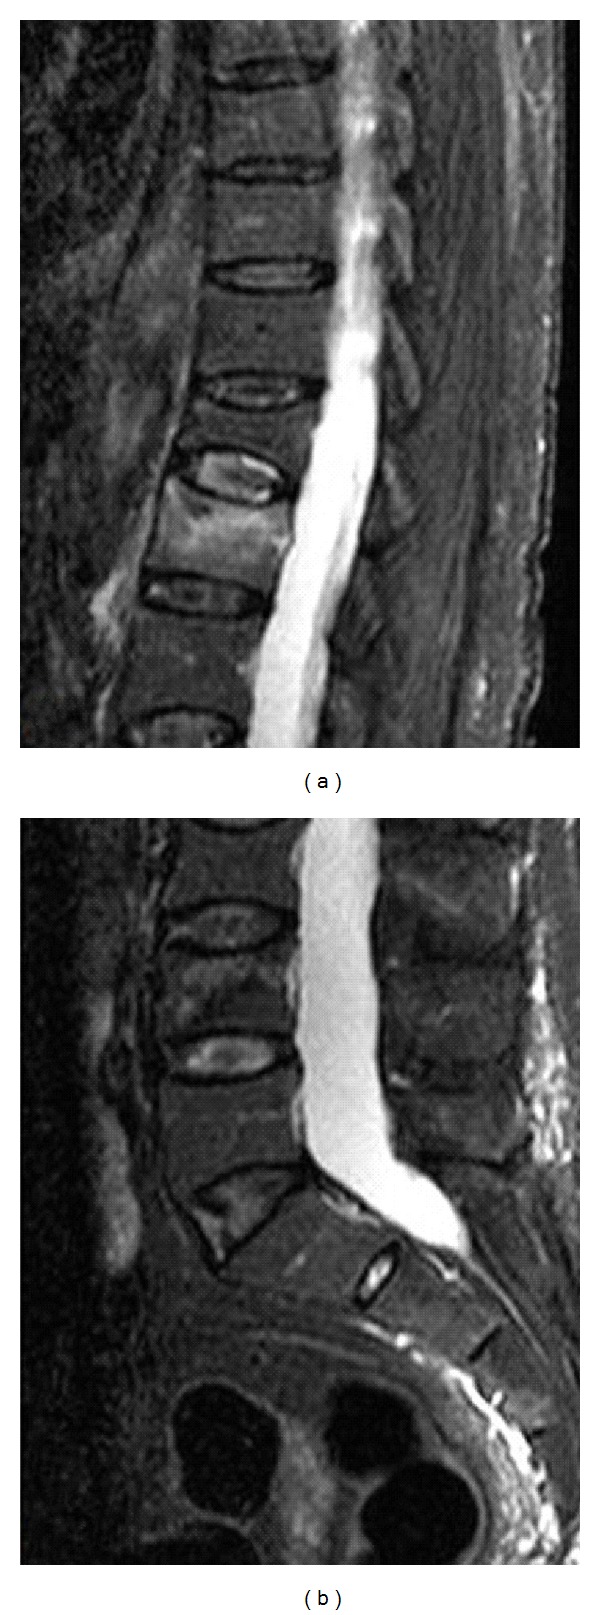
Sagittal STIR MR dorsal (a) and lumbar (b) image. MR image shows deformity of T12 vertebral body, which is chronic; there is no bone marrow edema. The recent fractures of L1 and L4 are much less deformed but have high signal from bone marrow edema.
